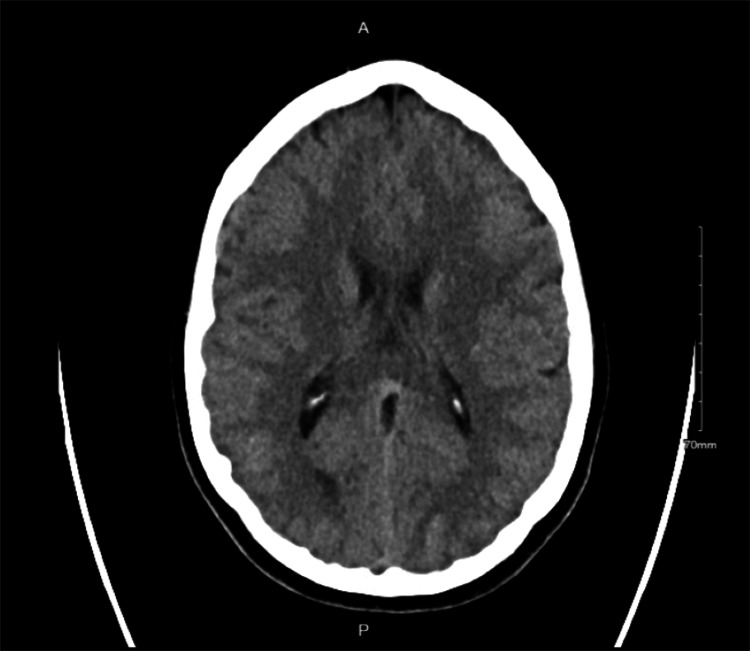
CT scan of the head showing normal findings CT: computed tomography
